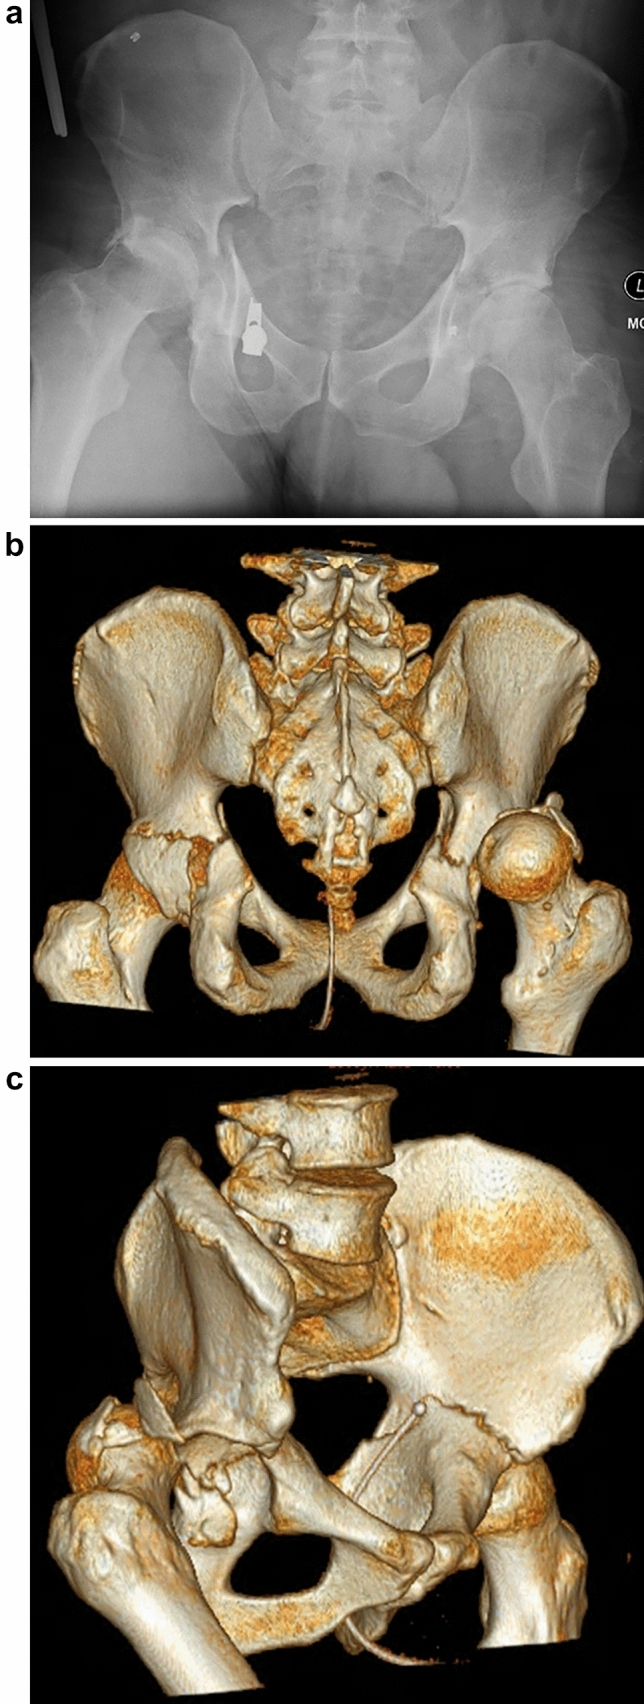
Anteroposterior pelvis radiograph (a) and posterior (b) and oblique (c) 3D surface-rendered CT reconstruction images showing a horizontal shear fracture with associated posterior wall fractures and right-sided posterior hip dislocation
